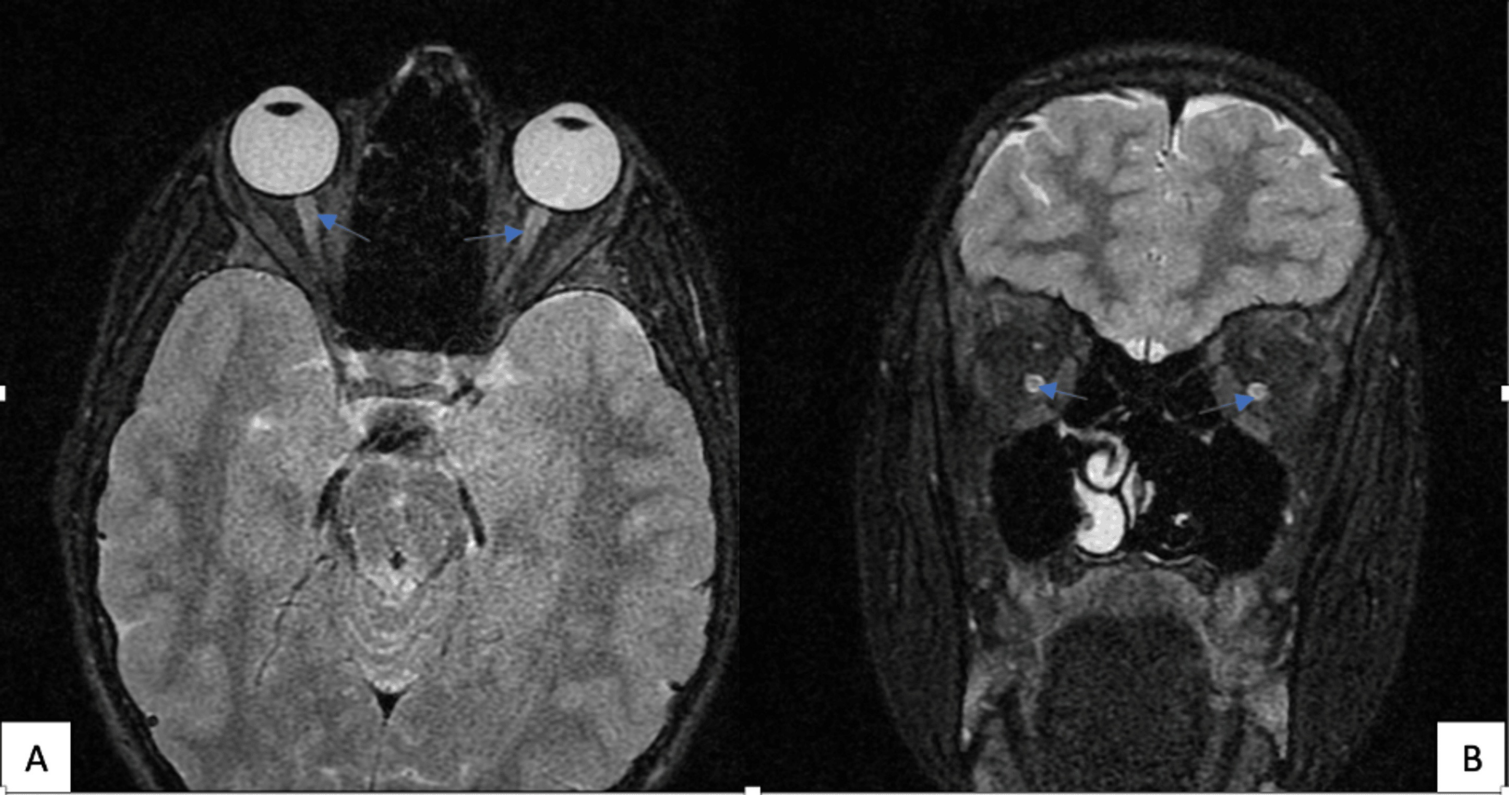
Bilateral optic atrophy in a patient with Wolfram syndrome (A and B) MRI showing symmetrical optic nerve atrophy (disk pallor) in axial and coronal views
